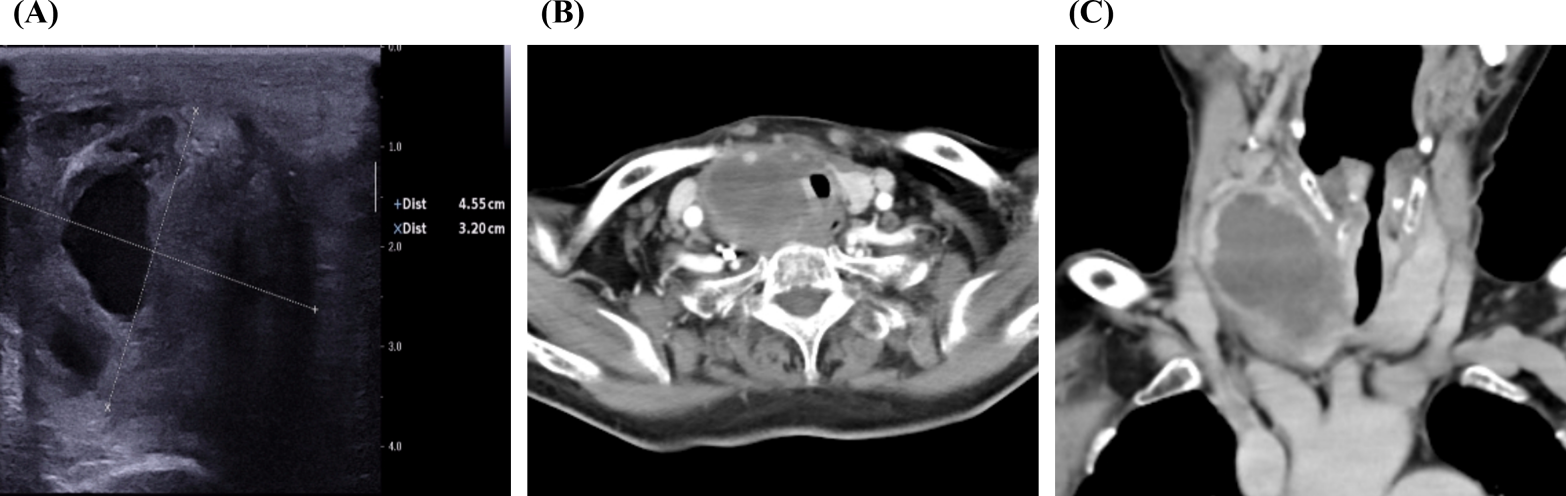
Typical image of imaging examination. (A) Ultrasound image of the tumor. (B) CT image of thyroid tumor in coronal section. (C) CT image of thyroid tumor in axial section.
